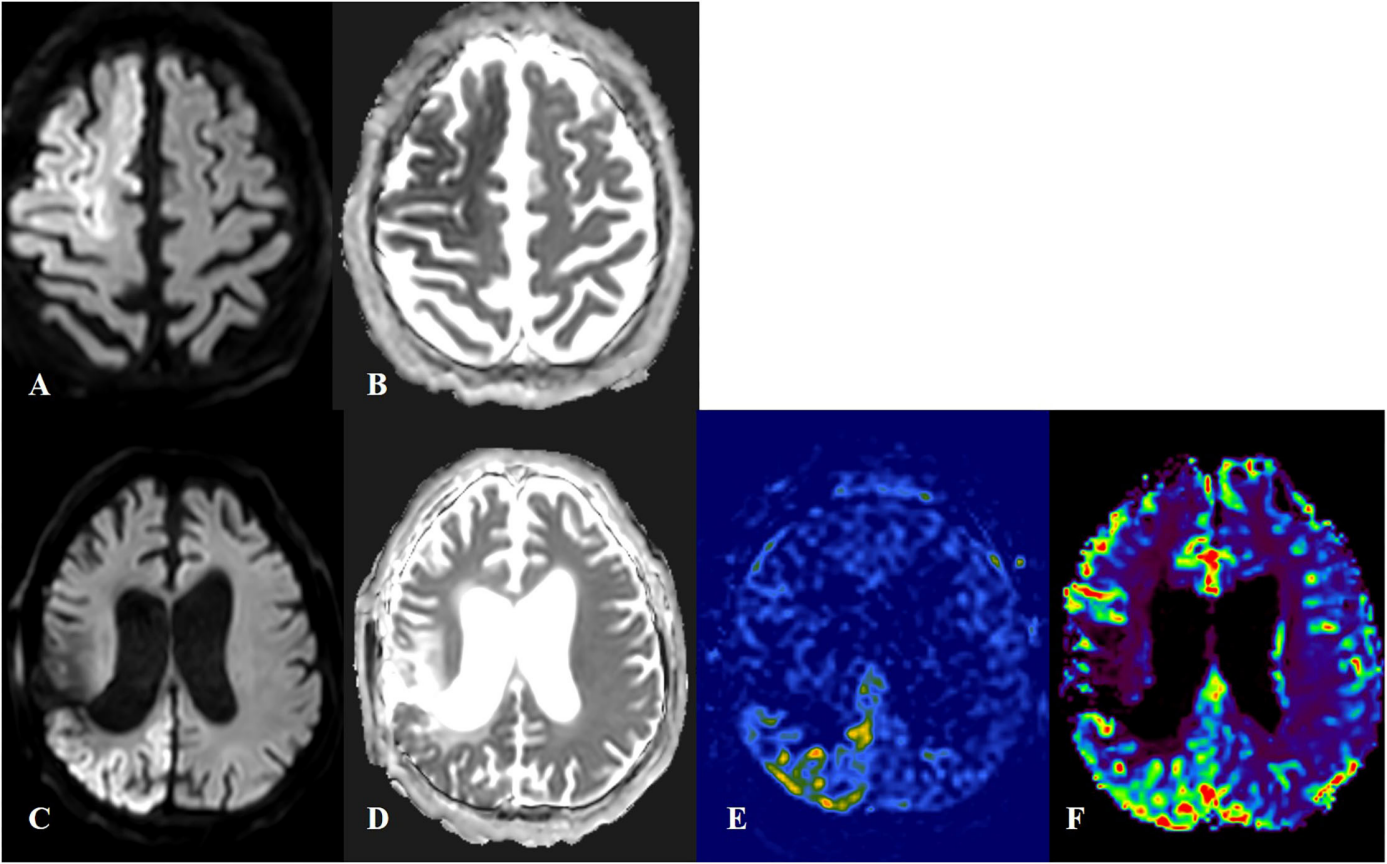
Cortical laminar necrosis in acute ischemic stroke (AIS) and status epilepticus (SE). First row: Patient with AIS in territories of right anterior and middle cerebral arteries, with an increased signal in diffusion-weighted imaging (DWI) (A) and decreased signal in apparent diffusion coefficient (ADC) (B). Quantification of DWI and ADC signals was done for the lesion side as compared to the contralateral healthy mirror side. The ratio for DWI lesion/healthy side was 1.70 and the ratio for ADC lesion/healthy side was 0.58. Second row: Patient with SE. The DWI-restricted lesion in the right parieto-occipital region (C,D) was suggestive of peri-ictal MRI abnormalities. In arterial spin labeling (E) and MRI-perfusion with a contrast substance (F), an ictal hyperperfusion in the right parieto-occipital region was observed. The ratio for DWI lesion/healthy side was 1.40 and the ratio for ADC lesion/healthy side was 0.84.
